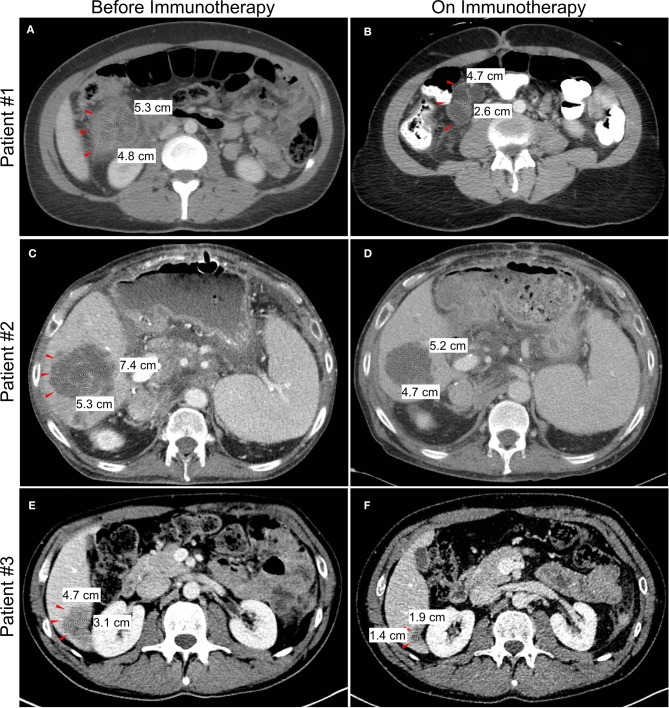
Changes in tumor or metastasis size by computed tomography (CT) imaging before and after initiation of checkpoint blockade immunotherapy. Representative imaging showing decrease in size of colon mass for patient #1 (A,B) and liver metastasis for patient #2 (C,D) and patient #3 (E,F).
